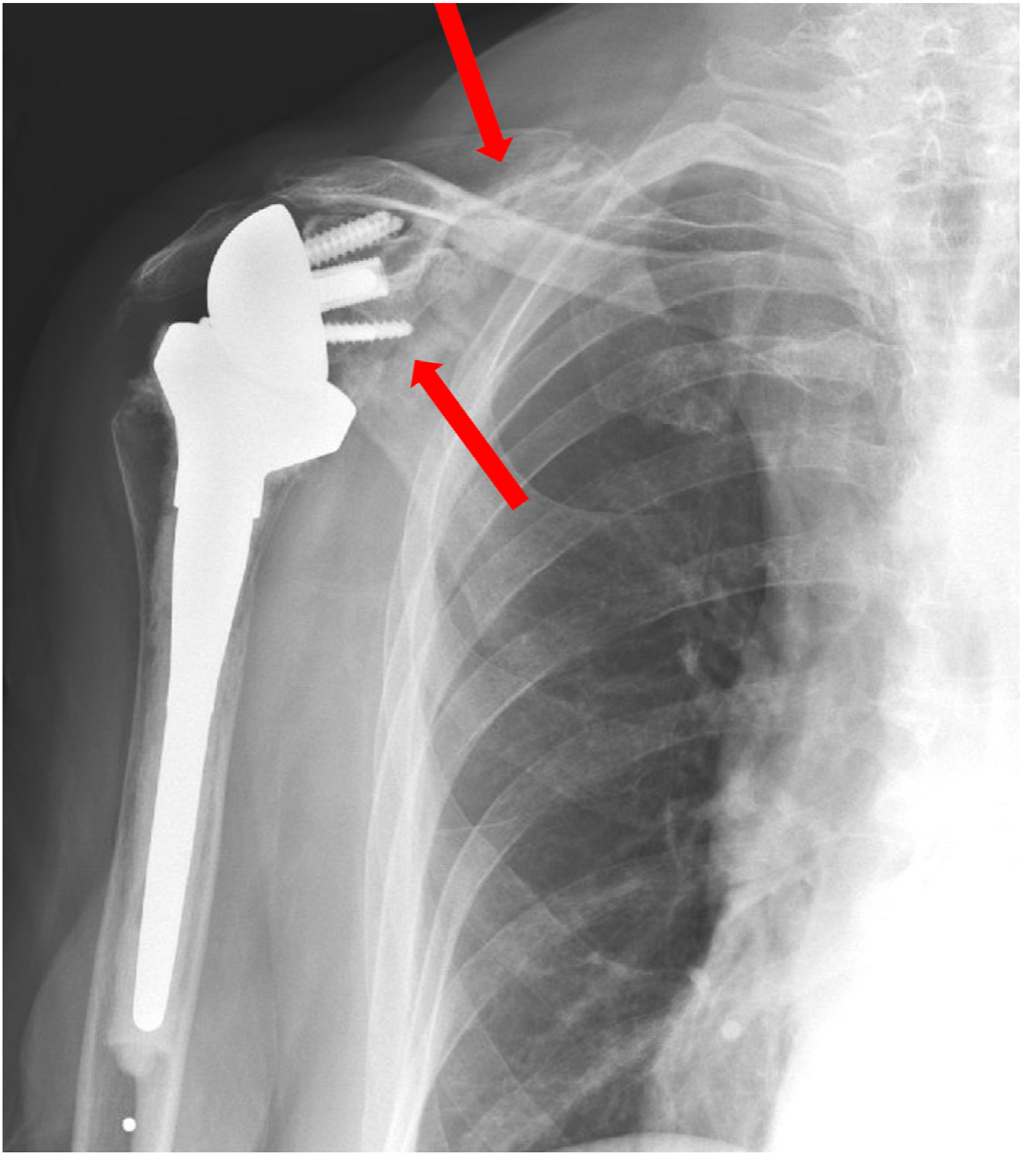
X-ray Grashey view demonstrating continued healing of the scapular body fracture.
